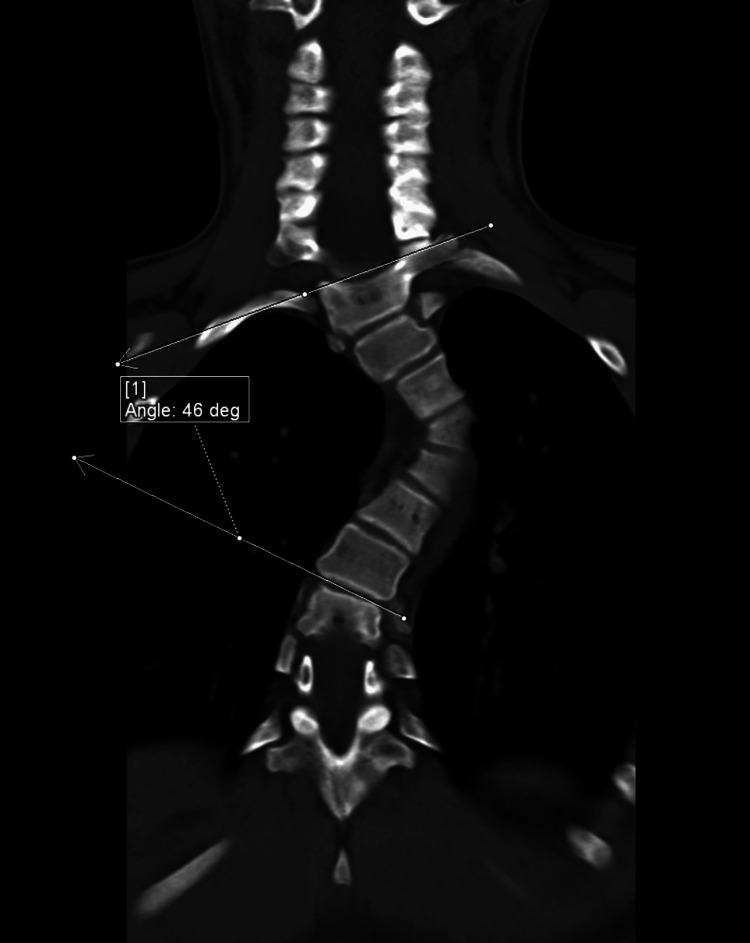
Reconstructed coronal CT image of the thoracic spine in bone kernel showing levocurvature of the thoracic spine with its apex at T4. The Cobb angle measured 46 degrees.
